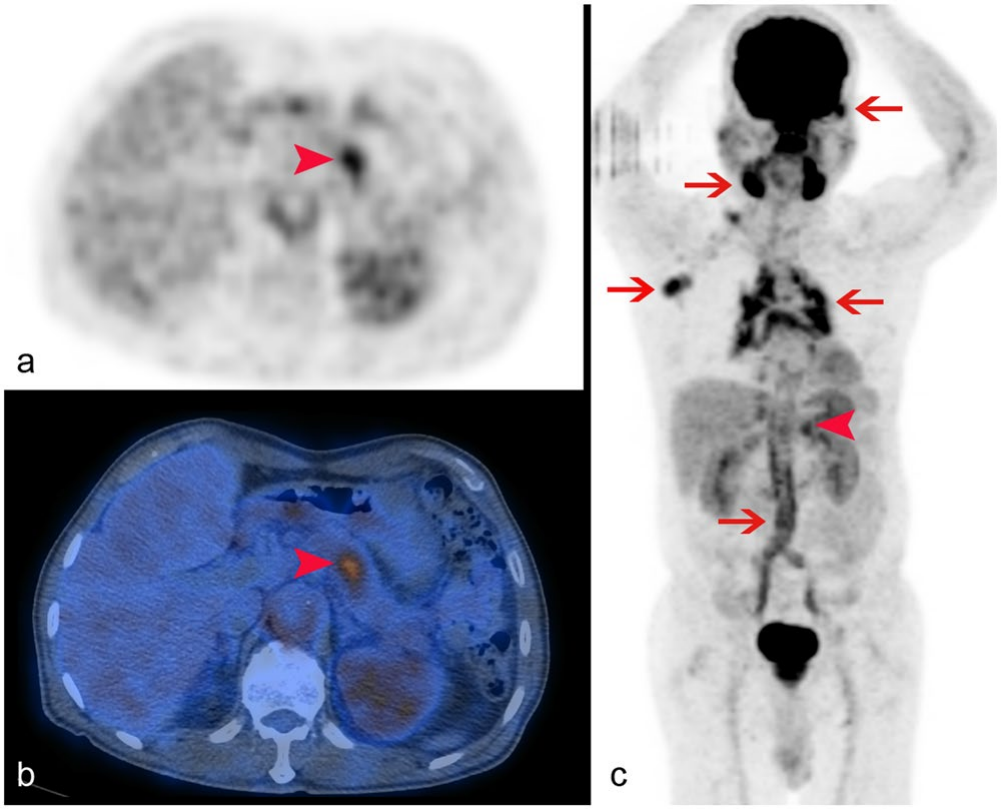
Autoimmune pancreatitis, focal type. Axial (a) and PET/CT fused (b) images showed a localized focus of intense hypermetabolism at the pancreatic body (arrowhead). The maximum intensity projection image (c) revealed more than two foci of extrapancreatic uptake, including the submandibular and left lacrimal glands, axillary and mediastinal nodes, and aorta to common iliac arteries (arrows). The primary pancreatic tumor exhibited a SUVmax of 5.1, and a high gray-level zone emphasis value of 125.4.
